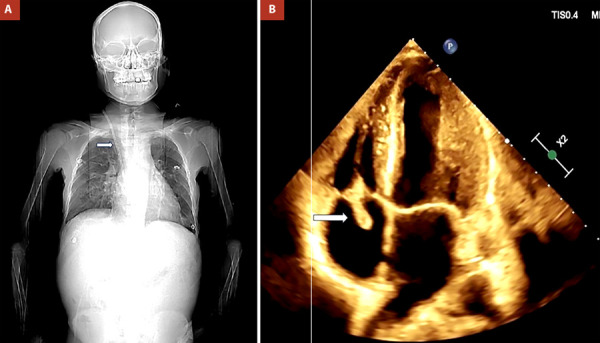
A. Coronal view of computed tomography shows tunneled central venous catheter (arrow). B. Transthoracic echocardiogram, demonstrating a pedunculated mass (20 x 16 mm) on the postero-lateral leaflet of the tricuspid valve (arrow).
